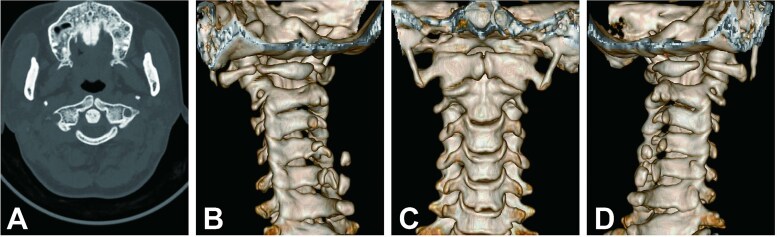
Cervical CT scan. (A) Axial CT image showing hypoplasia of the posterior arch and midline cleft of the anterior arch. (B–D) Three-dimensional reconstructed CT images showing atlas deformity from different views.
